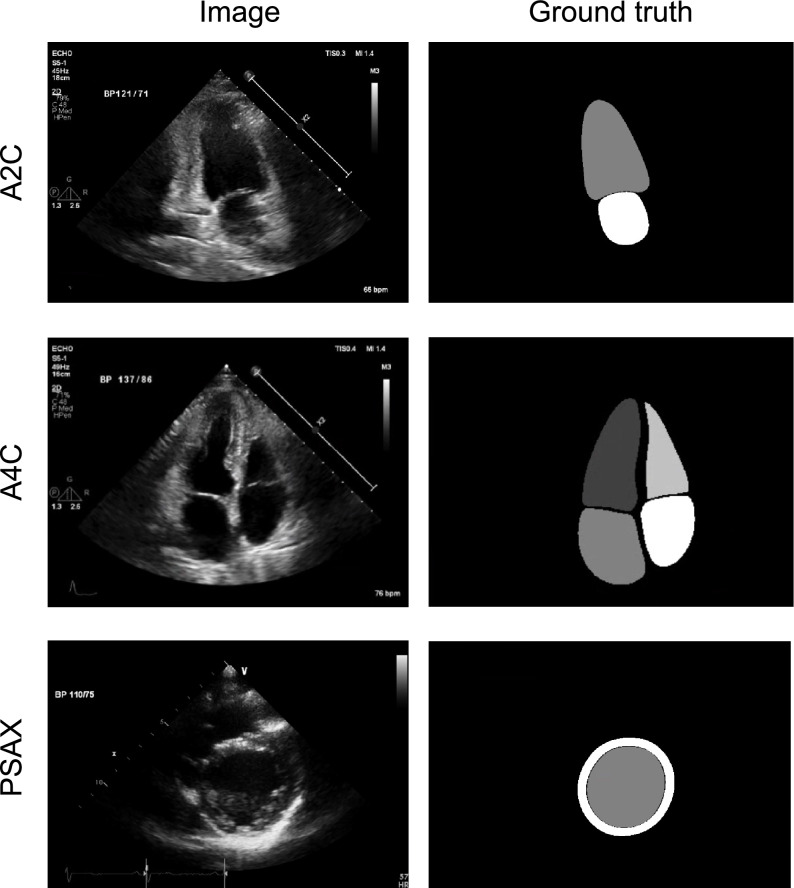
Representative echocardiography images and segmented cardiac chambers by experts
